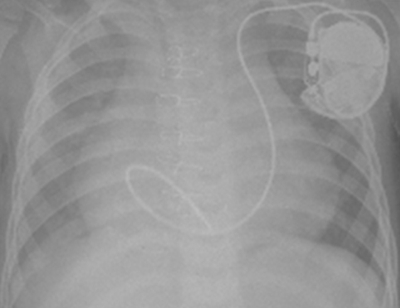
Chest X-ray shows transvenous active ventricular lead implantation via the persistent left superior vena cava and coronary sinus
